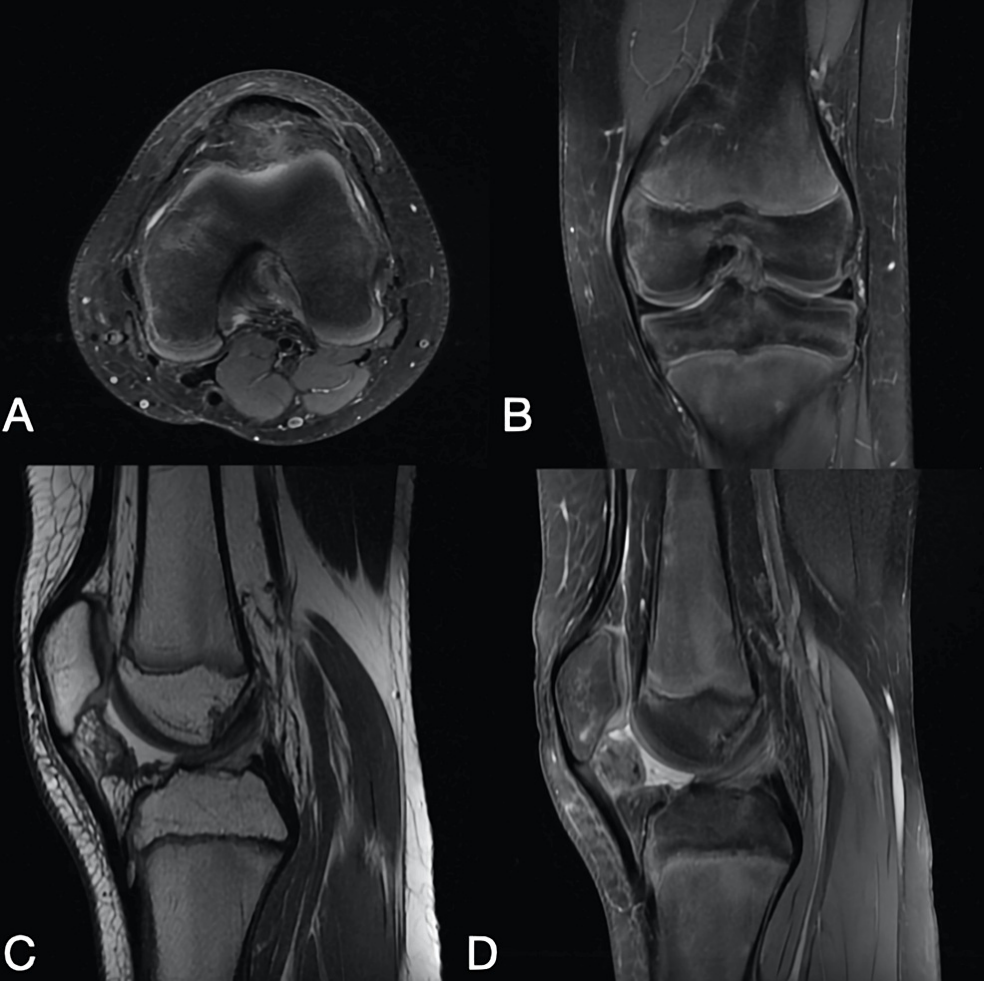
A: Four months postoperative left knee MRI in axial, B: Coronal, C and D: Sagittal planes shows a complete surgical removal of an ACL ganglion cyst.
